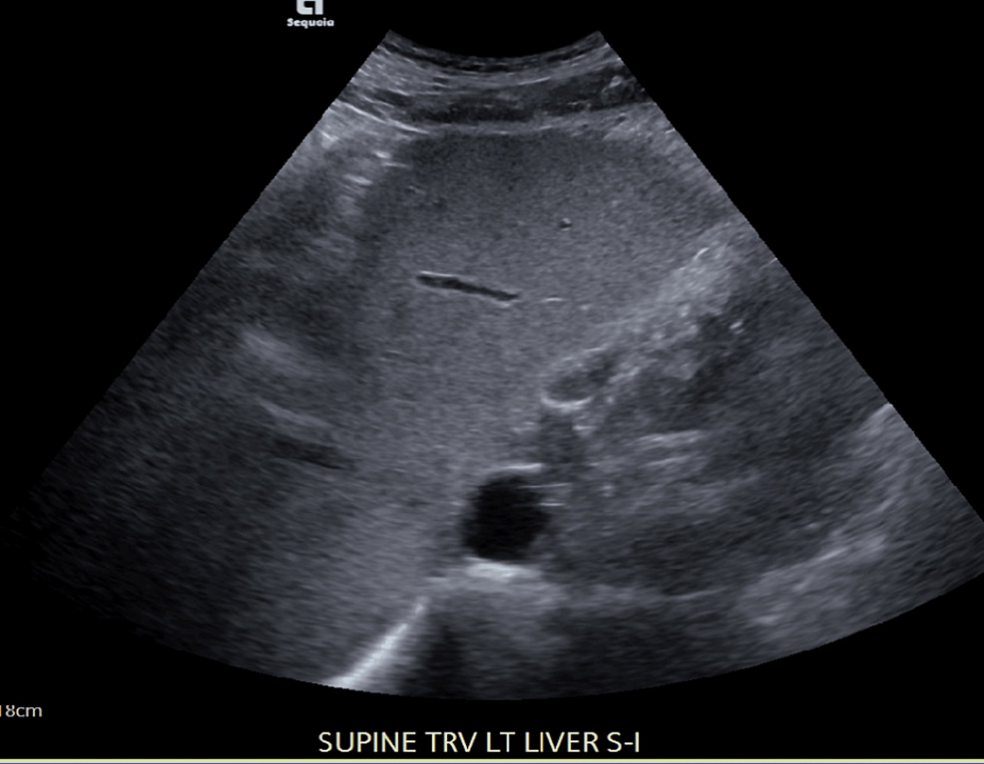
Ultrasound of the abdomen demonstrating a normal liver morphology
